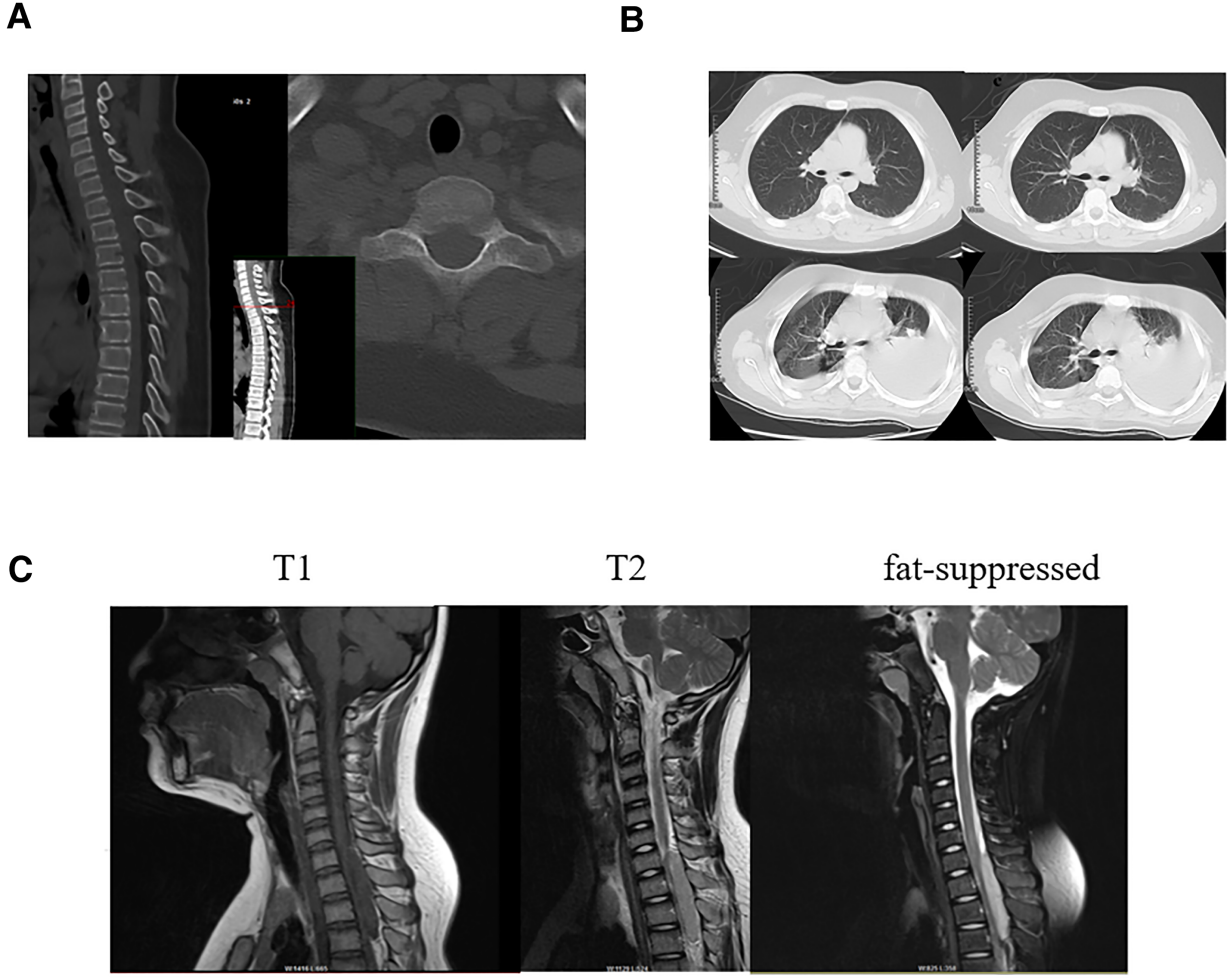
(A) Preoperative transverse and sagittal CT showed no bone destruction in the cervicothoracic junction. (B) Preoperative lung CT showed no obvious signs of tumor metastasis,Six months after the operation, lung CT showed multiple nodules in both lungs, and metastasis was considered. (C) T1-weighted, T2-weighted, and fat-suppressed sagittal MRI showed a long tubular mass in the spinal canal at the cervicothoracic junction, with a size of about 8 mm × 15 mm × 63 mm, and no abnormal signal vertebral body and appendage morphology.
